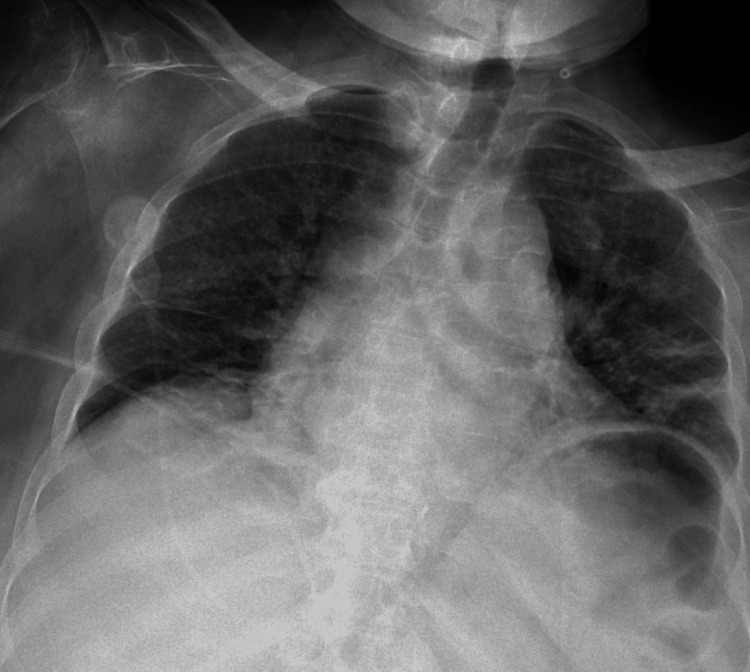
Chest Radiograph Demonstrating Moderate Bilateral Pulmonary Edema in the Setting of Sepsis and Volume Overload
